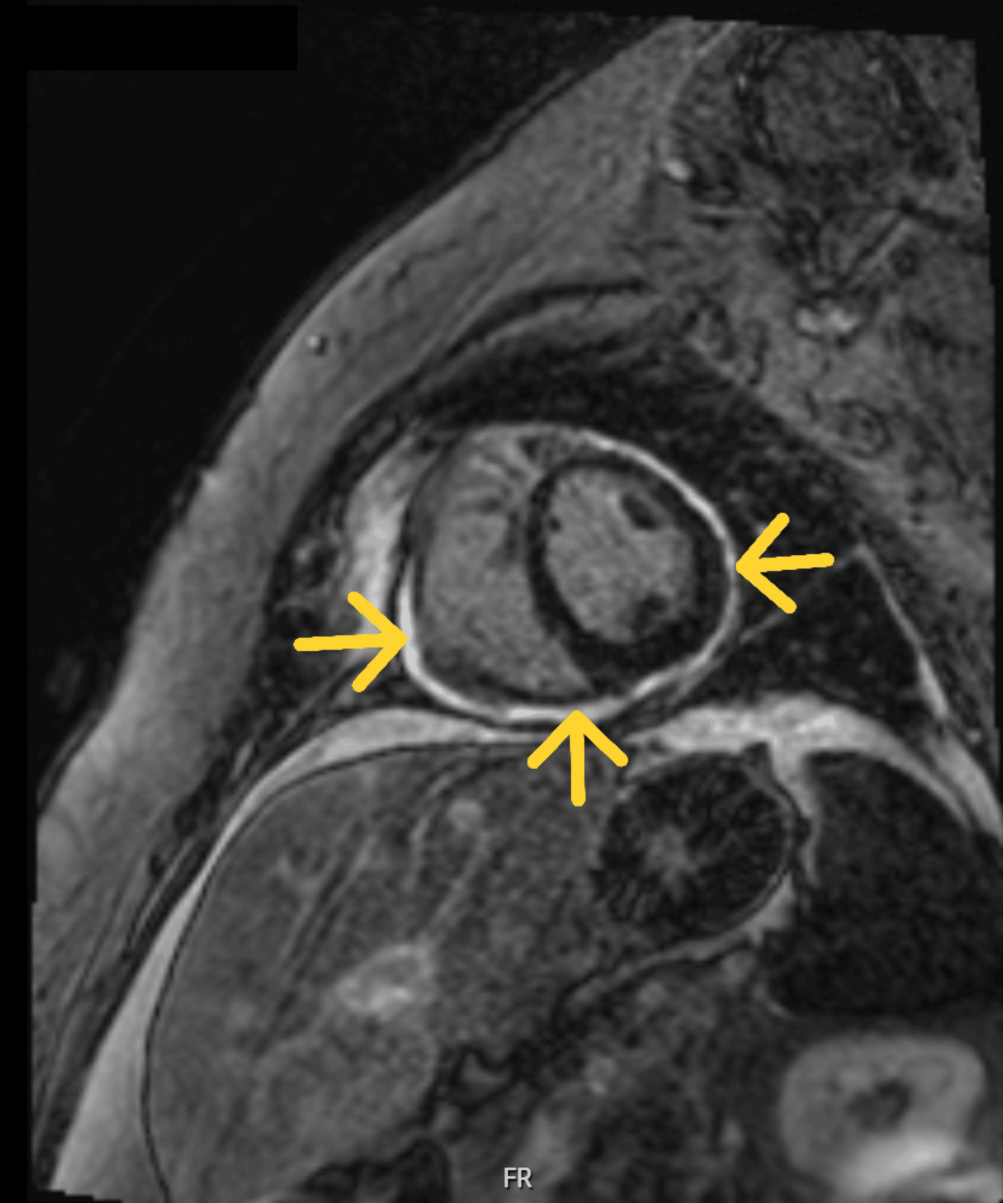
Cardiac MRI parasternal short-axis view showing pericardial thickening and late gadolinium enhancement of the pericardium (yellow arrows)
